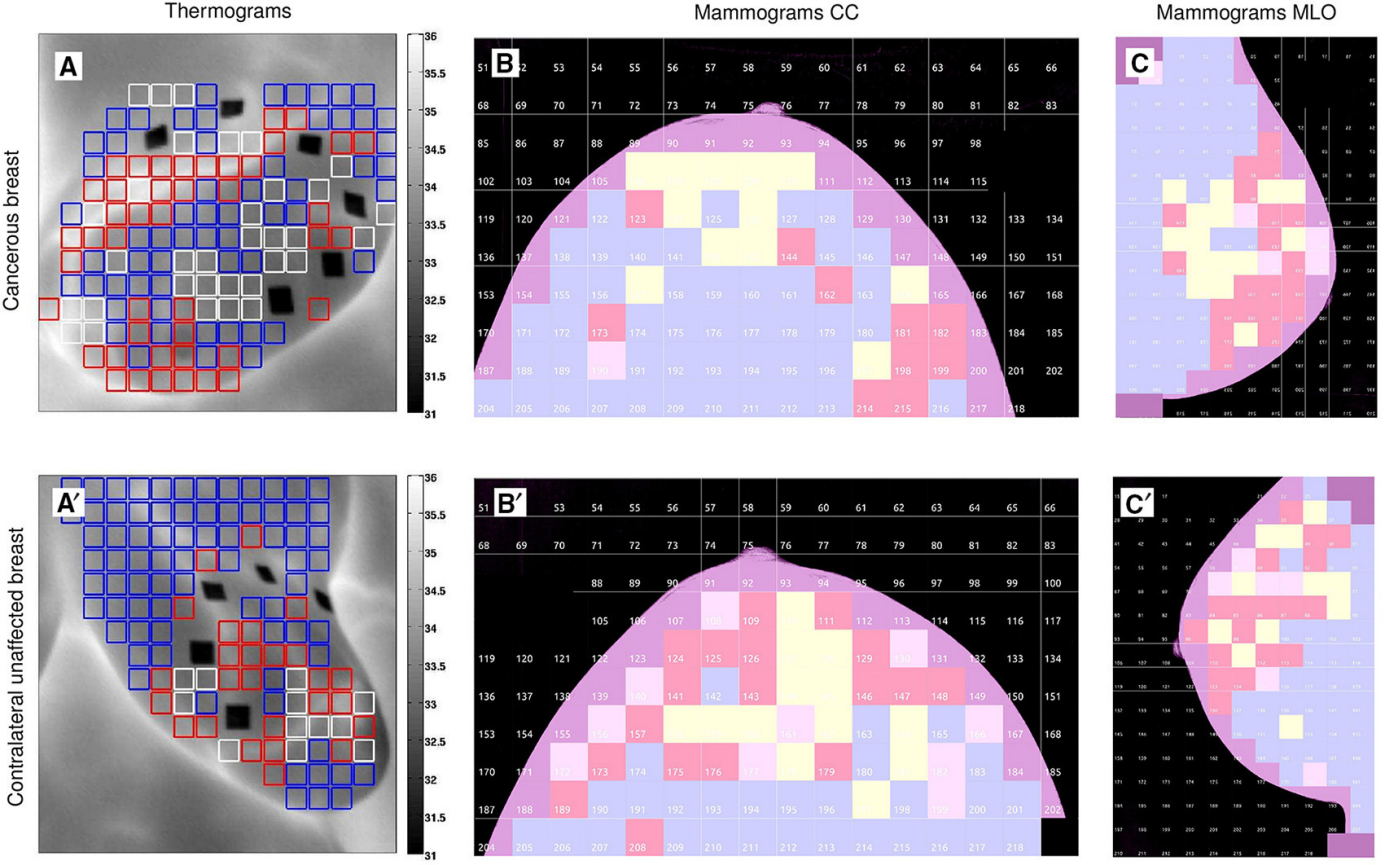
Wavelet-based multifractal segmentation of dynamic infrared thermograms and X-ray mammograms. Same as Figure 1 but for patient 7 (age 47): cancerous right breast (A–C) and contralateral unaffected left breast (A′–C′).
